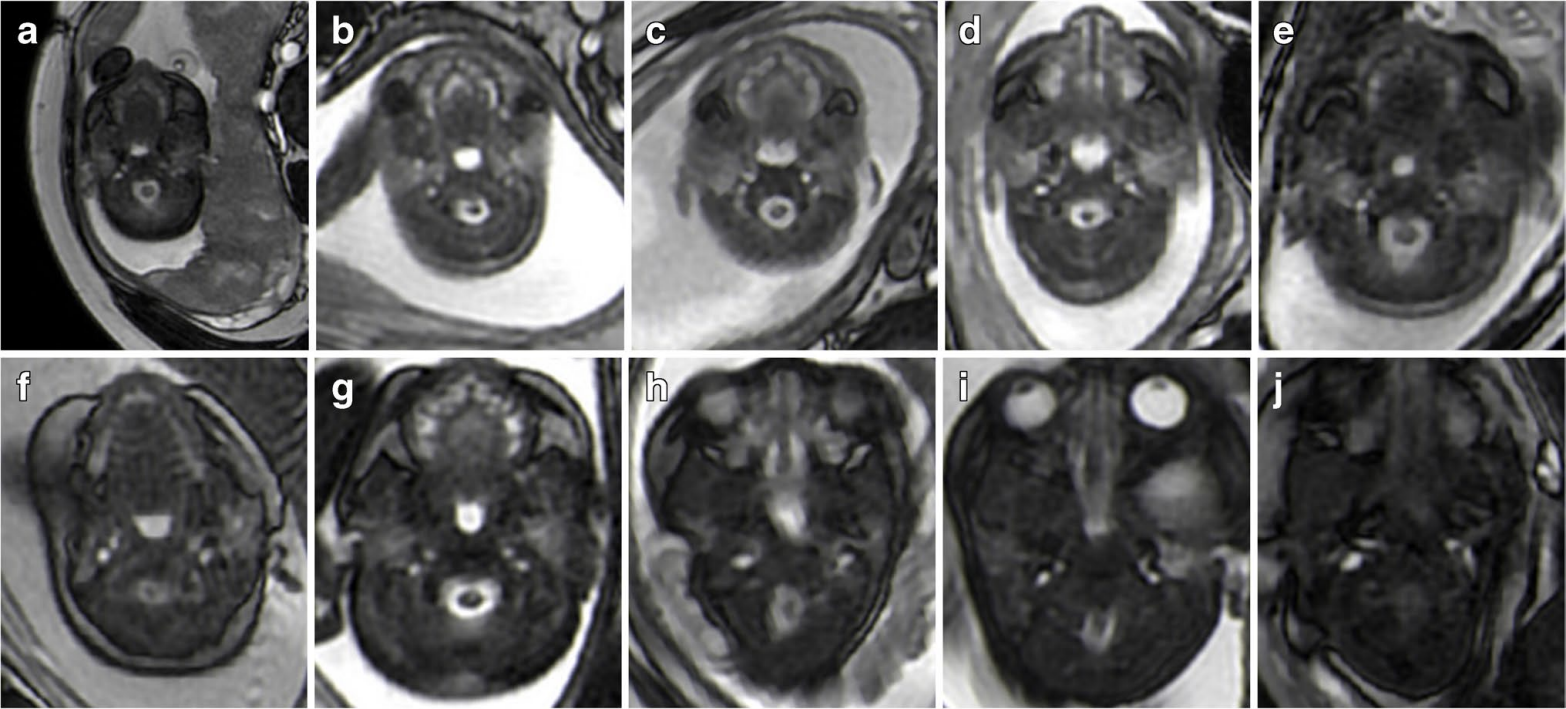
Axial balanced steady-state free precession magnetic resonance images of bilateral internal jugular veins of fetuses from 21–39 weeks of gestation. a 21-week-old female fetus. b 22-week-old male fetus. c 24-week-old male fetus d 28-week-old male fetus e 30-week-old female fetus f 32-week-old male fetus g 34-week-old female fetus h 36-week-old male fetus i 38-week-old male fetus j 39-week-old male fetus
